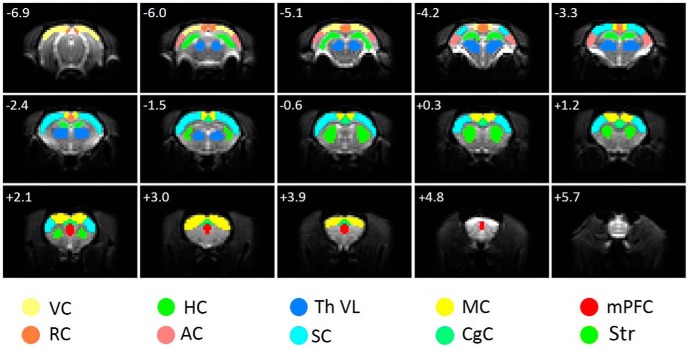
ROIs used for FC analysis. ROIs are overlaid on original spin-echo echoplanar imaging functional MRI images. AC, Auditory cortex; CgC, cingulate cortex; HC, hippocampus; MC, motor cortex; mPFC, medial prefrontal cortex; RC, retrosplenial cortex; SC, somatosensory cortex; Str, striatum; Th VL, ventral lateral thalamus; VC, visual cortex. Distance to bregma in millimeters is marked in the top left corner of each slice.
